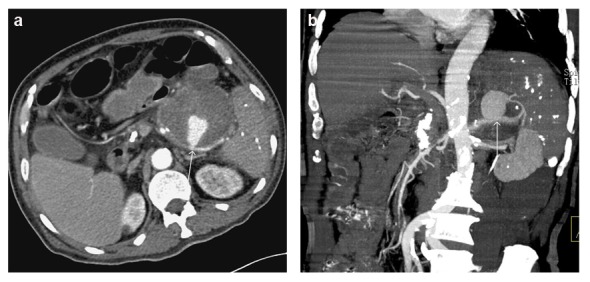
Axial and coronary reconstructed CT images showed splenic arterial pseudoaneurysm and giant pancreatic pseudocyst in the tail of the pancreas.
